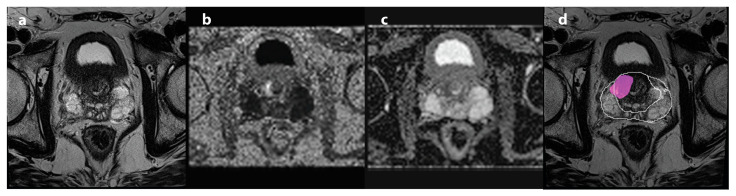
MRI examination obtained from a 70-year-old patient with an elevated PSA of 7.4 ng/mL. Image of the axial T2w sequence (a), the axial calculated b = 2000 s/mm^2^ DWI sequence (b), the axial ADC map (c), and the axial T2w sequences with the AI-generated map showing prostate and lesion segmentation (d). The combined 12-core TRUS guided fusion biopsy proved GS 3 + 4 prostate cancer only in targeted biopsy core of suspicious lesion. ADC = apparent diffusion coefficient, DWI = diffusion-weighted imaging, GS = Gleason score, TRUS = transrectal ultrasound.
